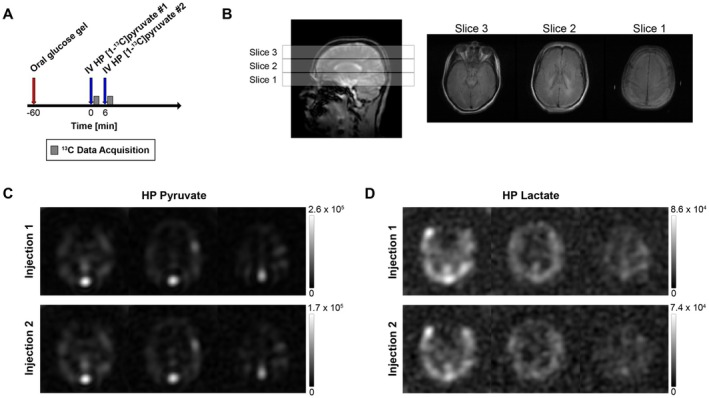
Metabolite‐interleaved spiral imaging of a healthy volunteer with two consecutive HP pyruvate. (A) Two samples of HP [1‐^13^C]pyruvate were injected to a 39‐years‐old male (participant ID: #9) subjected for ^13^C metabolite‐interleaved spiral imaging with a 6‐min interval. (B) ^1^H MRI of the prescribed axial slices for ^13^C imaging. Time‐accumulated ^13^C MRI of (C) HP pyruvate and (D) HP lactate maps from the corresponding slices.
